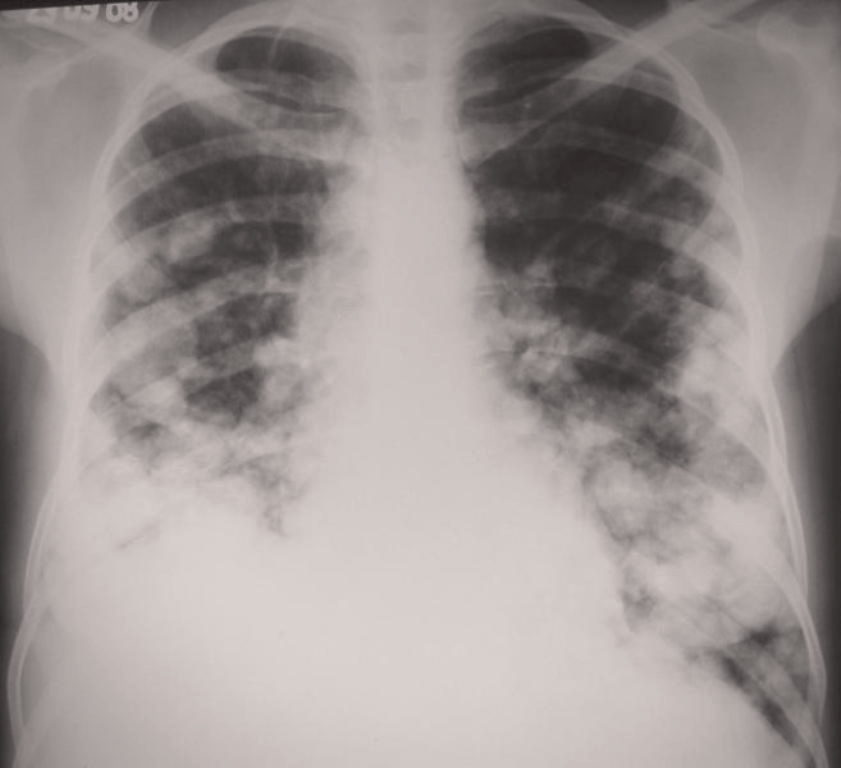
Chest X-ray showing multiple variable sized nodules predominating in the inferior areas of the lungs.
